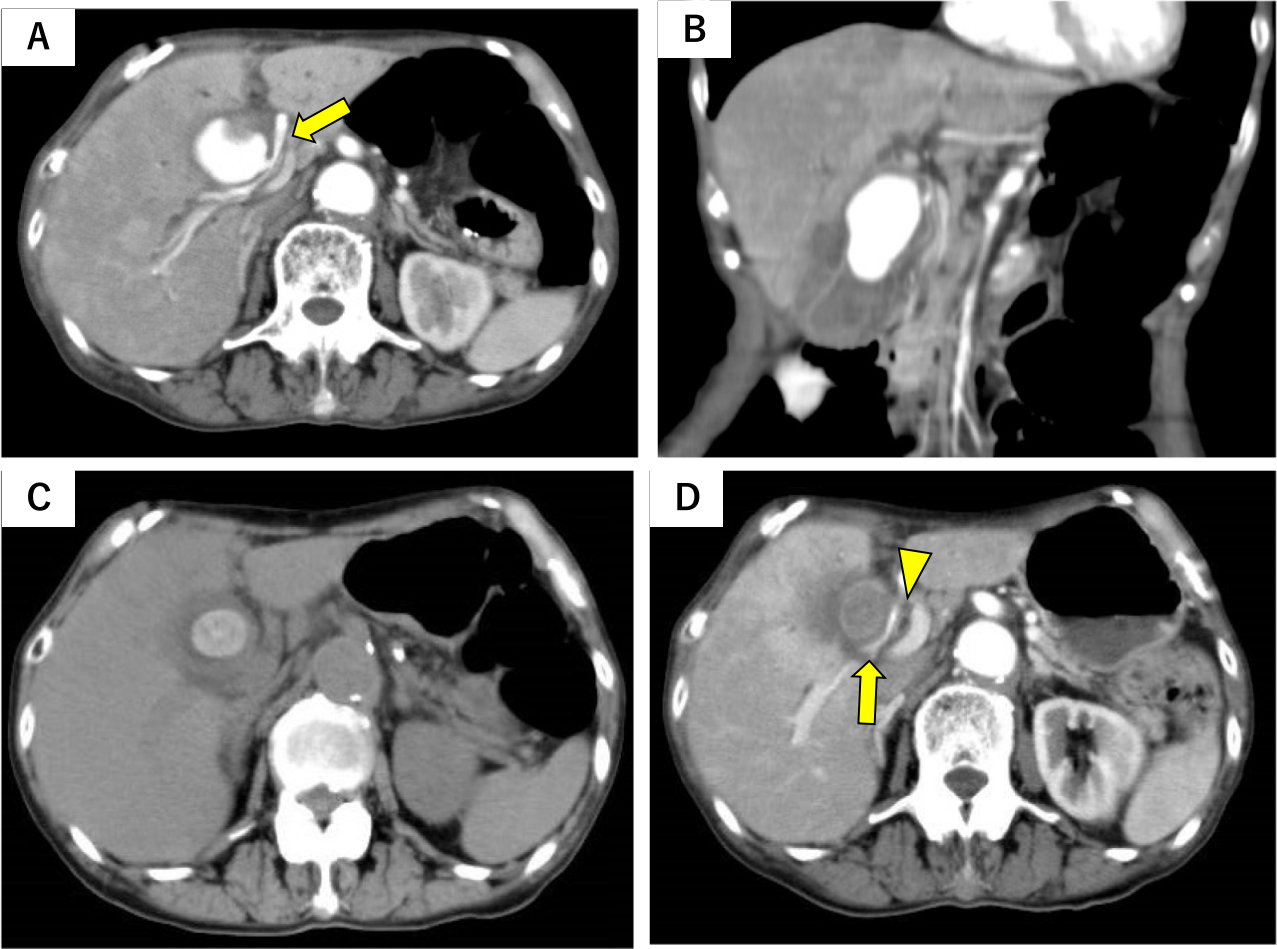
Enhanced abdominal computed tomography (CT) findings. Enhanced CT revealed a pseudoaneurysm in the gallbladder, which was suspected to be connected to the right hepatic artery or the cystic artery A (yellow arrow) axial view, B coronal view. Moreover, CT revealed a 20-mm gallstone in the neck of the gallbladder (C). The right hepatic artery (yellow arrow) runs ventral to the common bile duct (yellow arrowhead) and is in close proximity to the gallbladder (D)
